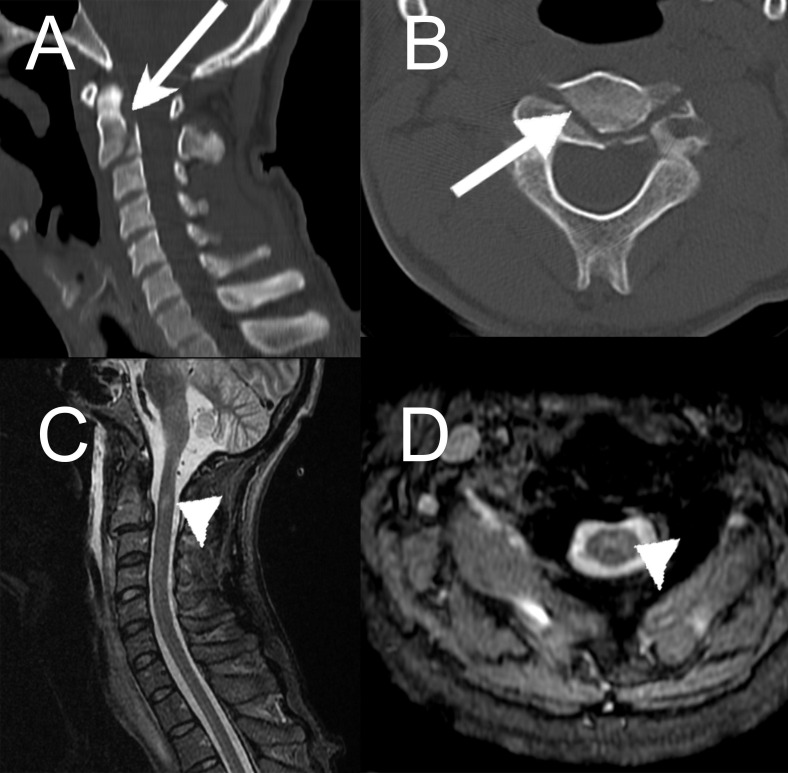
Case 2. Cervical spine computed tomography (CT) and magnetic resonance imaging (MRI) scans. (A, B) A C2-C3 complex fracture (Anderson 3) with traumatic spondylolisthesis (Hangman 2) is observed (arrows). (C, D) A traumatic spondylolisthesis C2-C3 with a C2 contusion with marked myelopathy is demonstrated (arrowheads).
